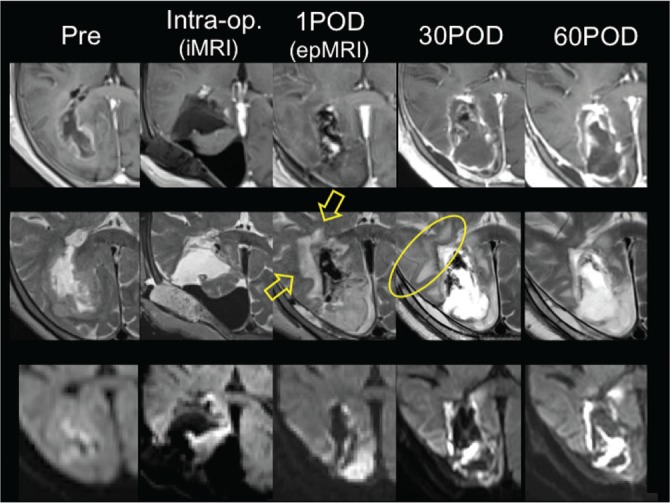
Gadolinium (Gd)-enhanced T_1_-weighted imaging (T_1_WI) (upper), T_2_WI (center) and diffusion-weighted imaging (DWI) (lower) of a representative carmustine (BCNU) wafer-implanted case (76 y.o. woman, initially diagnosed with glioblastoma) with obvious early postoperative high-intensity area on T_2_WI (ep-T_2_-HIA) expansion with a depth of 17.6 mm (yellow arrows) in the adjacent parenchymal area without markedly restricted diffusivity. The ep-T_2_-HIA changes to delayed cerebral edema 30 days after the surgery (yellow circle).
